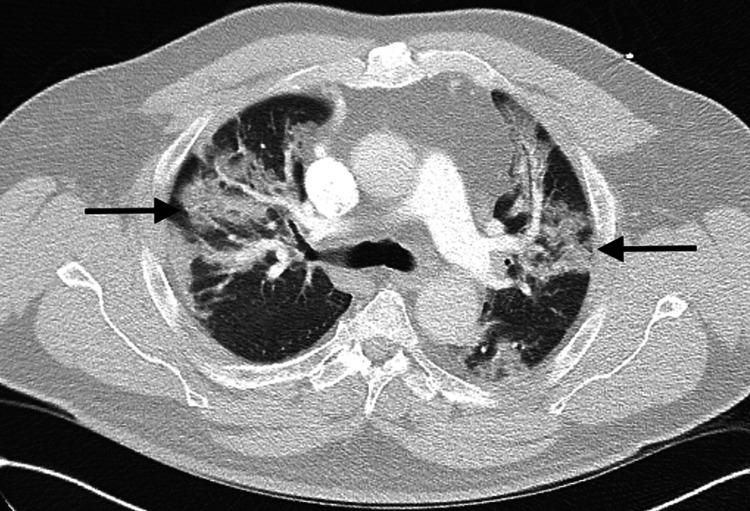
Chest CT demonstrating extensive bilateral ground glass infiltrates (arrows).
